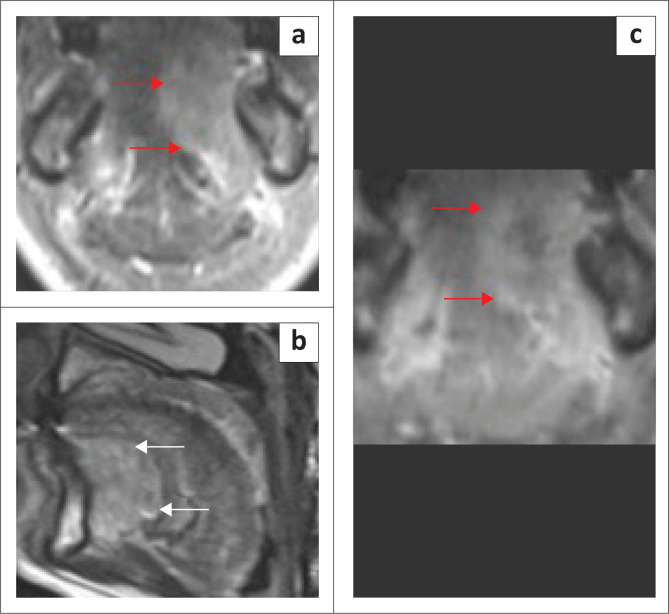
Left anterior floor of the mouth bulky small cell carcinoma (stage T4) in an elderly patient, invading the extrinsic tongue muscles. T2-weighted short-tau inversion recovery (T2W-STIR) coronal (a) and sagittal T2 (b) demonstrate the mildly hyperintense, bulky tumour. The T1 contrast-enhanced fat-suppressed image (c) reveals an ill-defined, inhomogeneously enhancing tumour.
